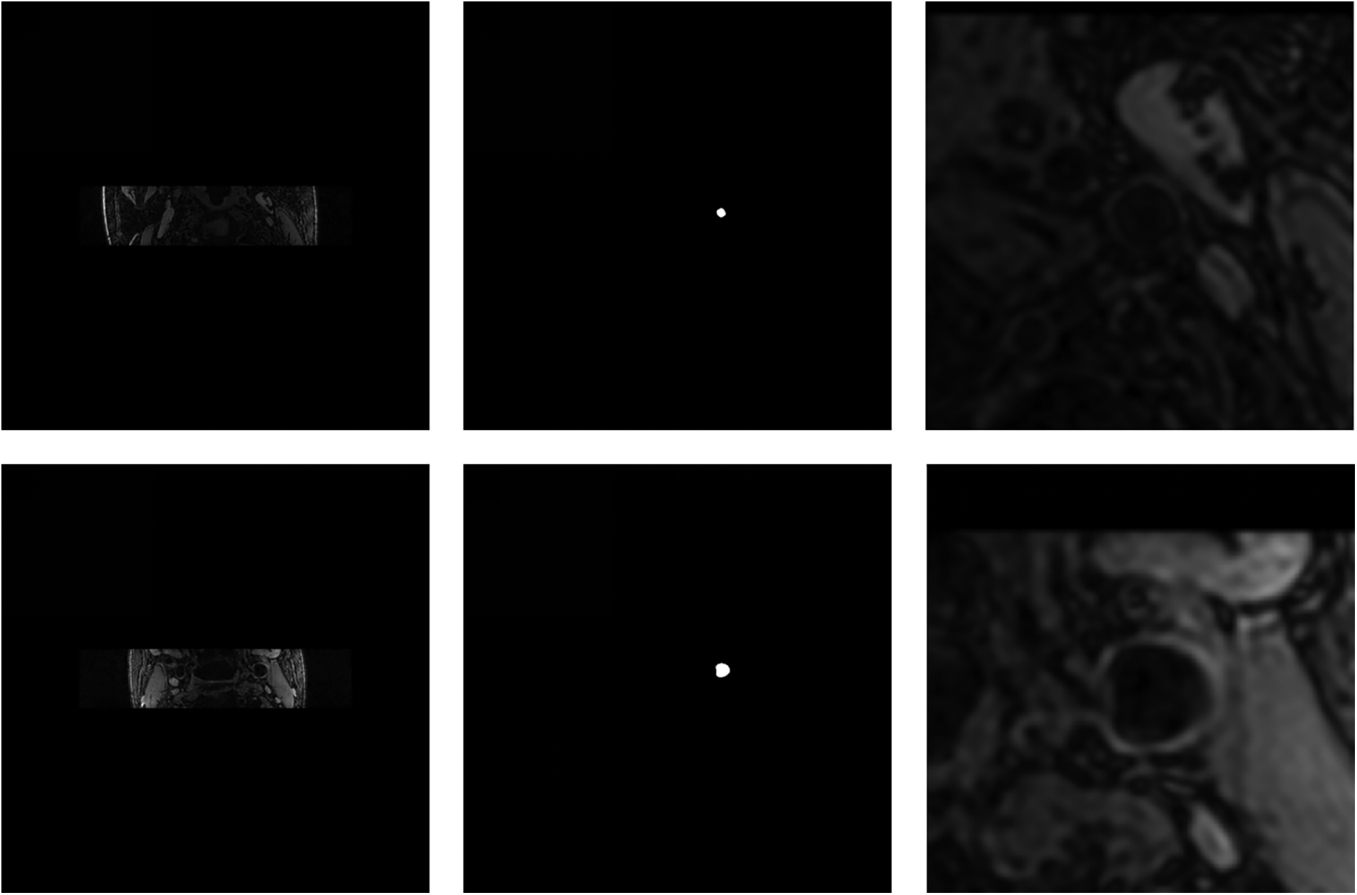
Visualization of the coarse segmentation. The first column are the original-resolution MRI images, the middle column are the centers of gravity estimated by coarse segmentation, and the last column are local patches obtained after passing through the vascular center-of-gravity positioning model.
